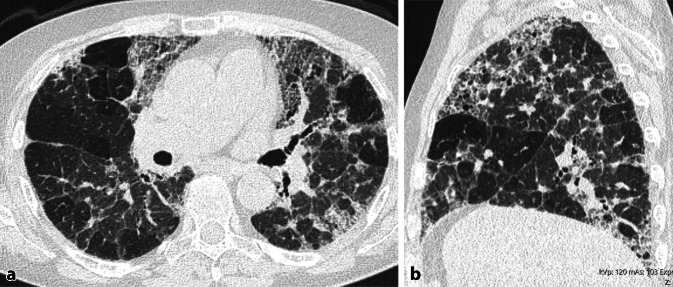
Fibrotische exogen-allergische Alveolitis (EAA) mit viel Inflammation. a Axiale und b sagittale multiplanare Reformation (MPR) einer nativen High-Resolution-Computertomographie (HRCT). Oberlappenbetonte Fibrose erkenntlich an irreguläre Retikulationen und Traktionsbronchiektasen. Zudem diffus verteiltes Milchglas und Air-Trapping. Beachte auch das Drei-Dichte-Zeichen
